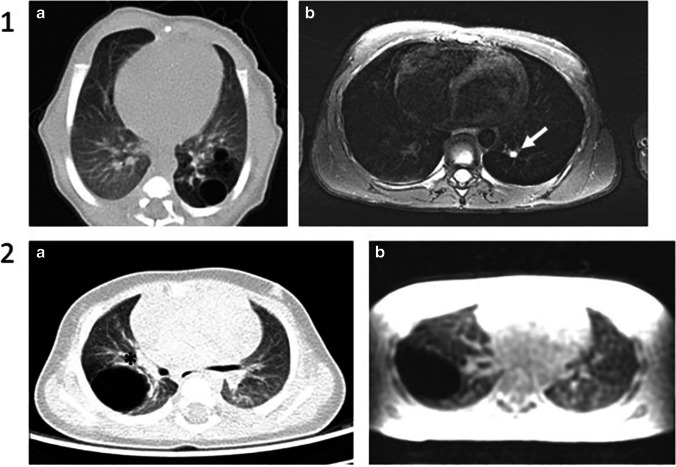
Example images of changed appearance of CLA between postnatal CT and school-age MRI. Patient 1: axial postnatal CT showing an air-filled multicystic CPAM and BA in the left lower lobe (a) and an axial T2-weighted PROPELLER image at school-age showing only BA in the left lower lobe (arrow) (b). Patient 2: axial postnatal CT showing an air-filled CPAM surrounded by lesion-associated parenchymal atelectasis (asterisk) (a) and an axial SPGR expiratory image at school-age showing an air-filled CPAM without lesion-associated parenchymal abnormalities (b)
